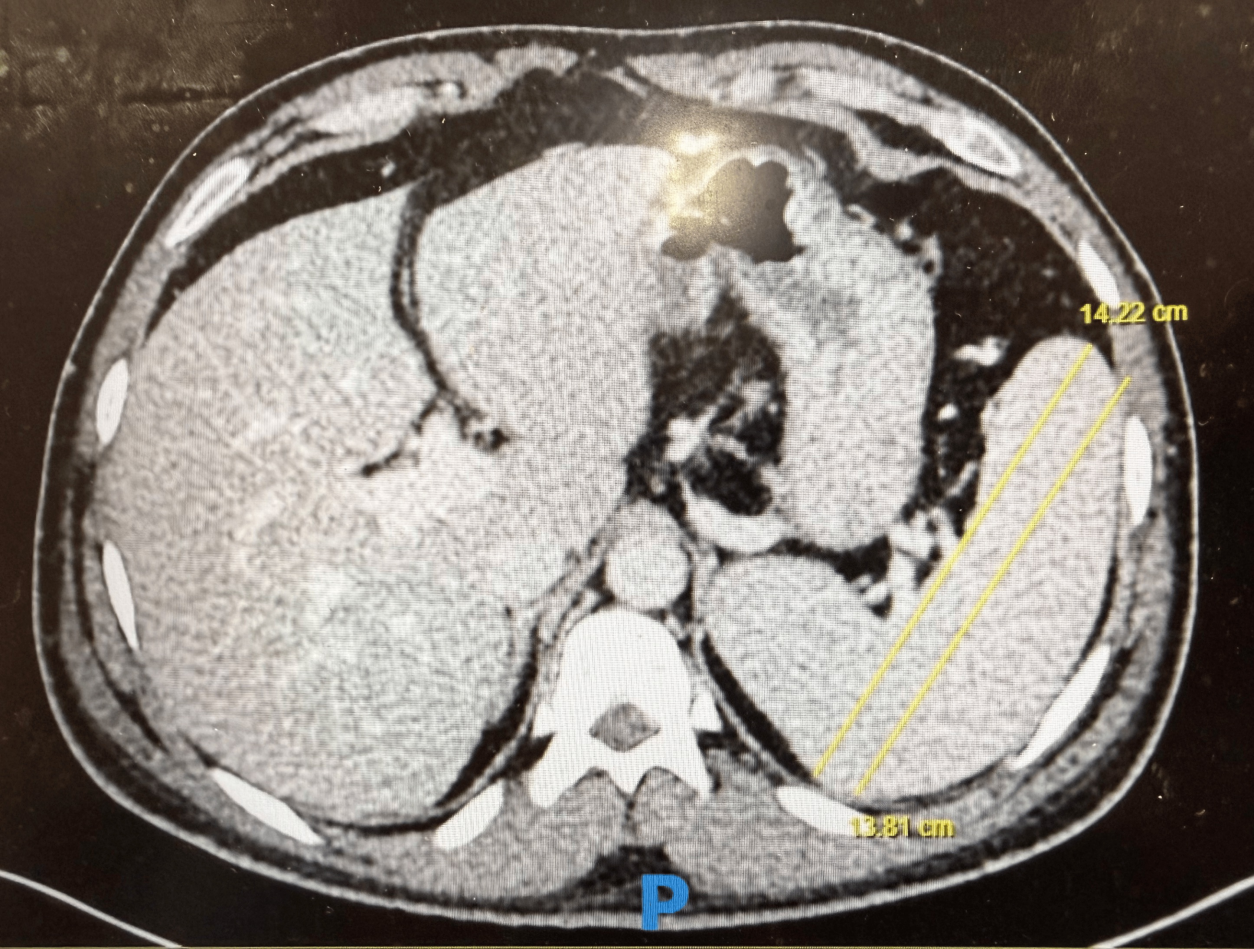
Contrast-enhanced CT of the abdomen showing splenomegaly, with splenic length measurements of 13.81 cm and 14.22 cm in two different planes.
